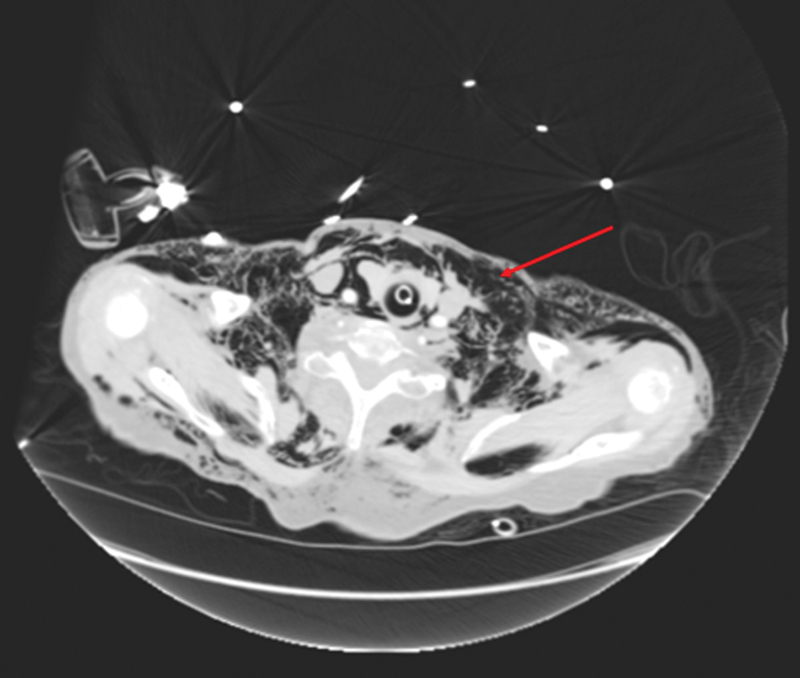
Axial computed tomography image of subcutaneous emphysema in the neck (red arrow).
